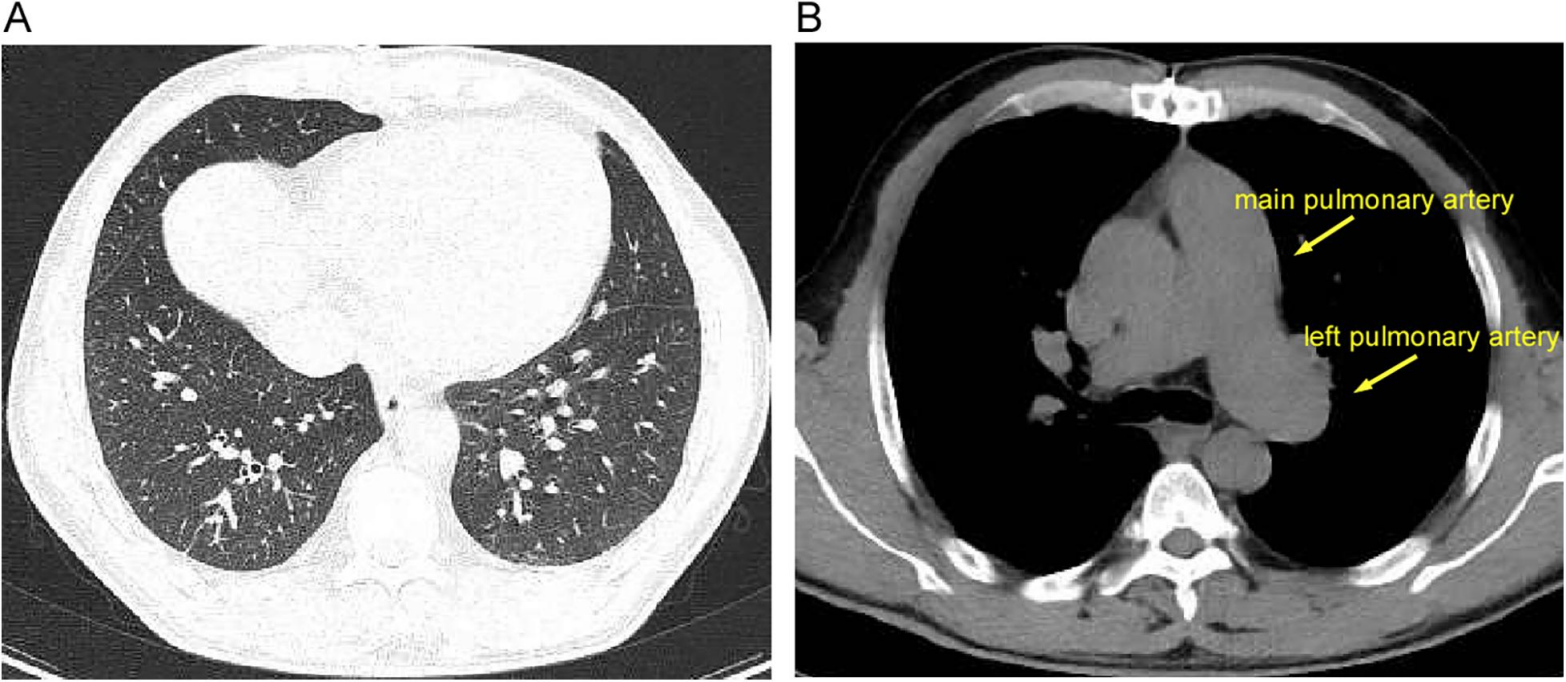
Postoperative chest CT at 3-month follow-up. (A) Chest CT, lung window, demonstrating resolution of the previously noted bilateral pulmonary infiltrates. (B) Chest CT, mediastinal window, showing reduced dimensions of the main pulmonary artery (1.83 cm) and its left branch (3.12 cm) (yellow arrows) compared to the preoperative study (see Figure 2B). CT, computed tomography.
